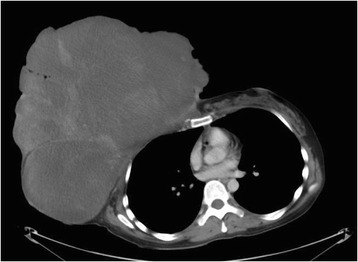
Contrast-enhanced computed tomography findings. The tumor measured 20 × 28 × 28 cm and was segmented. Free air is visible. The boundary between the tumor and the pectoralis major muscle is unclear. The axillary lymph nodes were mildly enlarged, with a maximum diameter of 10 mm on the right side. No distant metastases are noted
